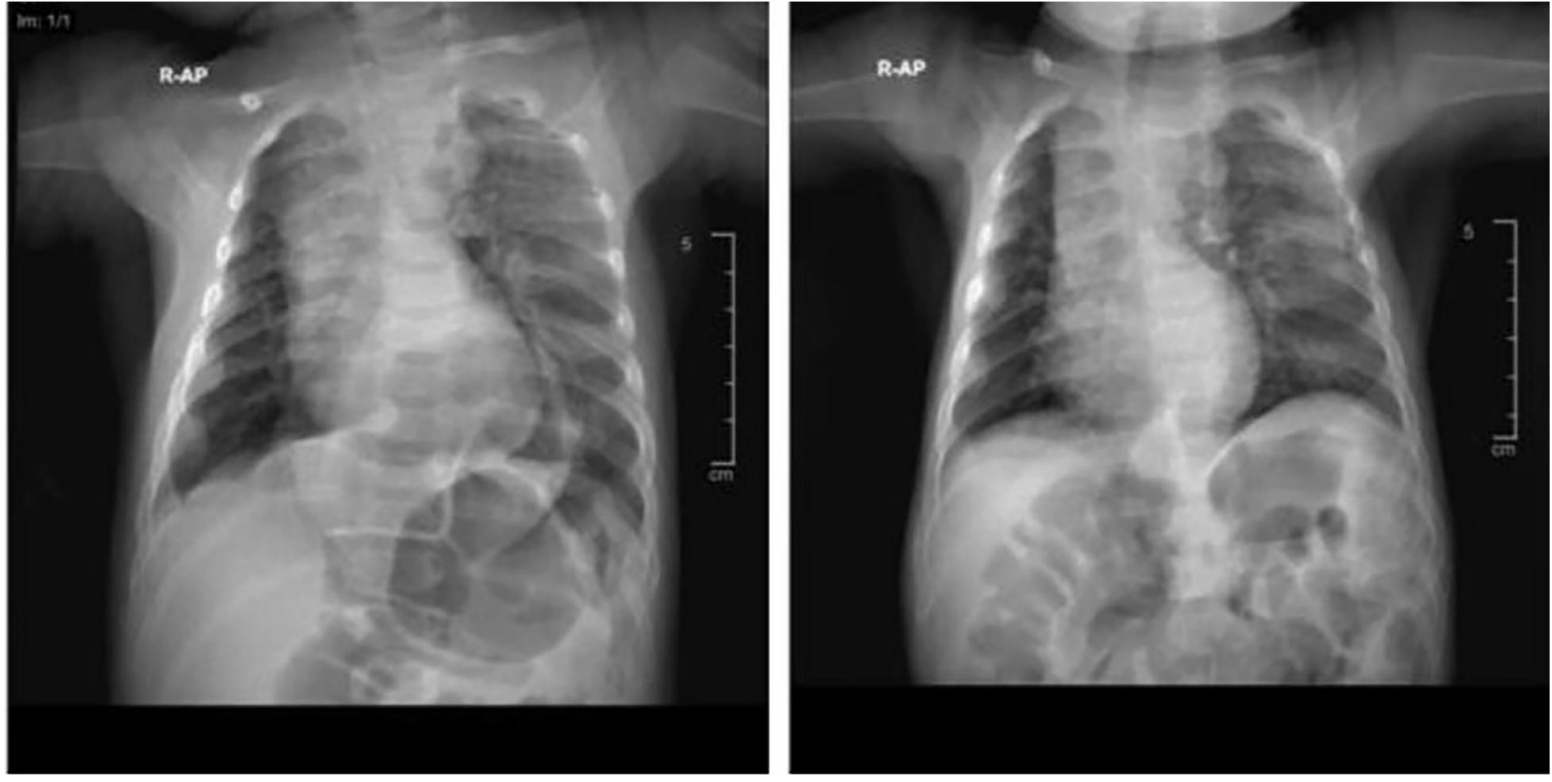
Anteroposterior chest X-rays of the patient (A) at the time of diagnosis and (B) 2 months postoperatively.
